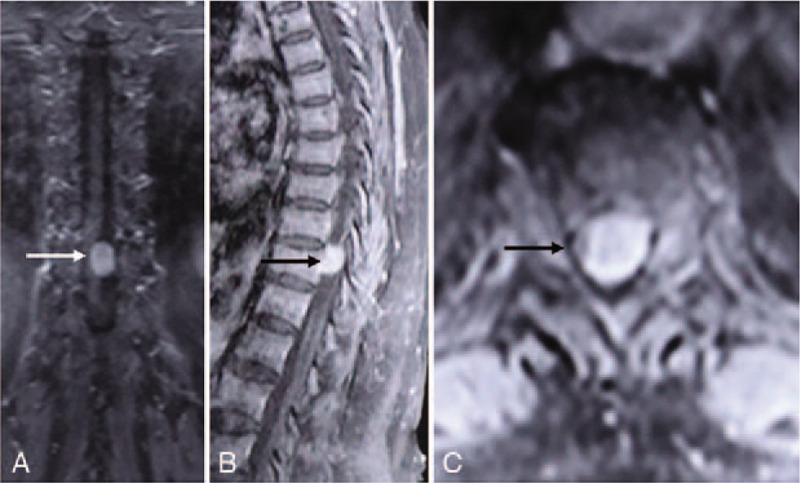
Dynamic contrast-enhanced magnetic resonance imaging scan showing a lateral intradural extramedullary tumor at the T11 level with a diffuse enhanced signal. The coronal plane revealed that the spinal cord was pushed to the right side by the mass (A); the ventral basis of the mass has unclear border with the anterior spinal canal, thus suggesting an adhesion in the area (B).
